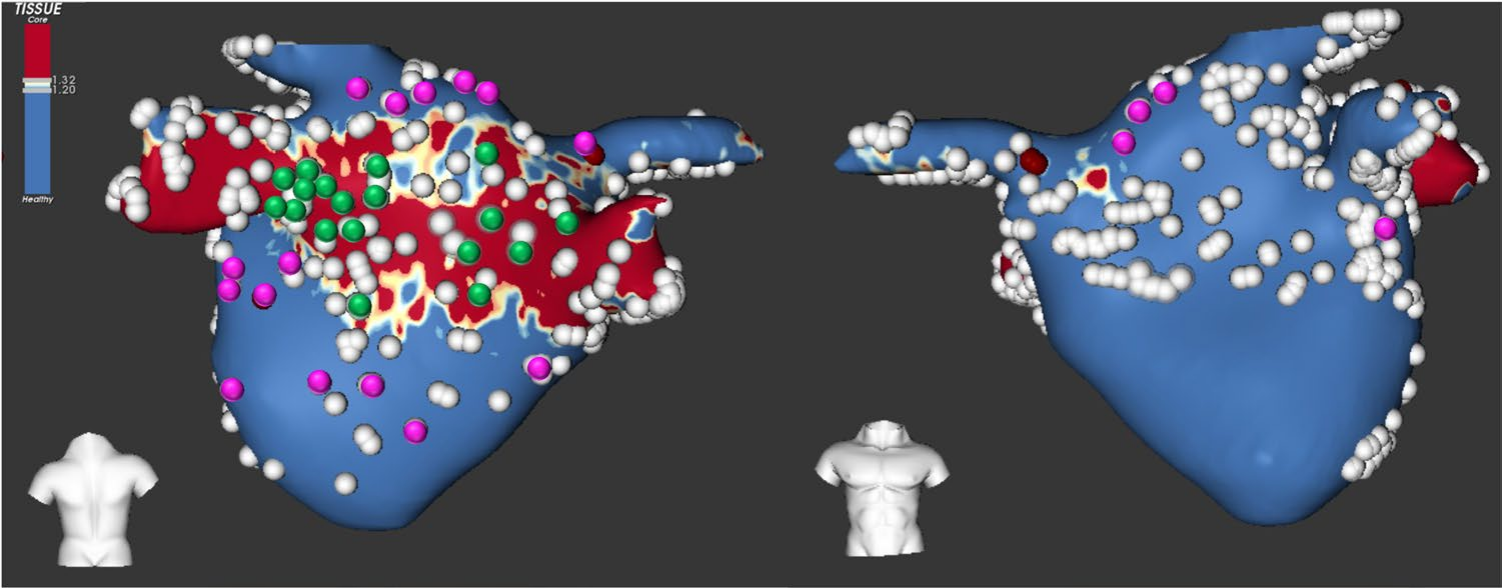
Posteroanterior (left) and anteroposterior (right) views of color-coded 3D mesh of MRI (showing dense fibrosis in red and healthy tissue in blue) generated by ADAS 3D co-registered with all EAM mapping points provided by CARTO 3 (gray). The 38 mapping points selected over fibrotic and non-fibrotic areas to compute EIGDR and bipolar indices are highlighted in green and magenta, respectively
